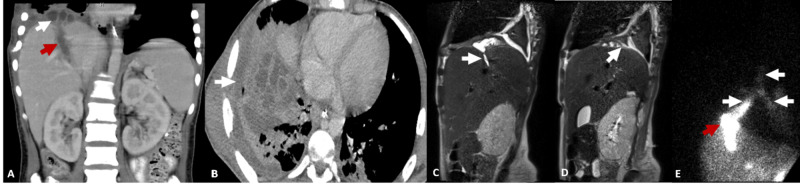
A 16-year-old male with history of penetrating injury (gunshot) to the right upper quadrant and right lower hemithorax complicated by biliary leak, diaphragmatic injury, and bilomas. (A) Coronal contrast-enhanced CT shows a fluid filled track (red arrow) and subdiaphragmatic multiloculated fluid collection (white arrow). (B) Axial contrast-enhanced CT shows a subdiaphragmatic multiloculated fluid collection (white arrow). (C) Sagittal Single Shot Fast Spin ECHO (SSFSE) images show a biliary tract extending to subdiapragmatic fluid collection (white arrow) and (D) communication between the subhepatic fluid collection and pleural cavity (white arrow). (E) Anterior planar image of hepatobiliary scintigraphy shows a focal area of increased activity in the subdiaphragmatic region, consistent with biloma (red) and abnormal activity in the bronchial tree, consistent with bronchobiliary fistula (white arrows).
